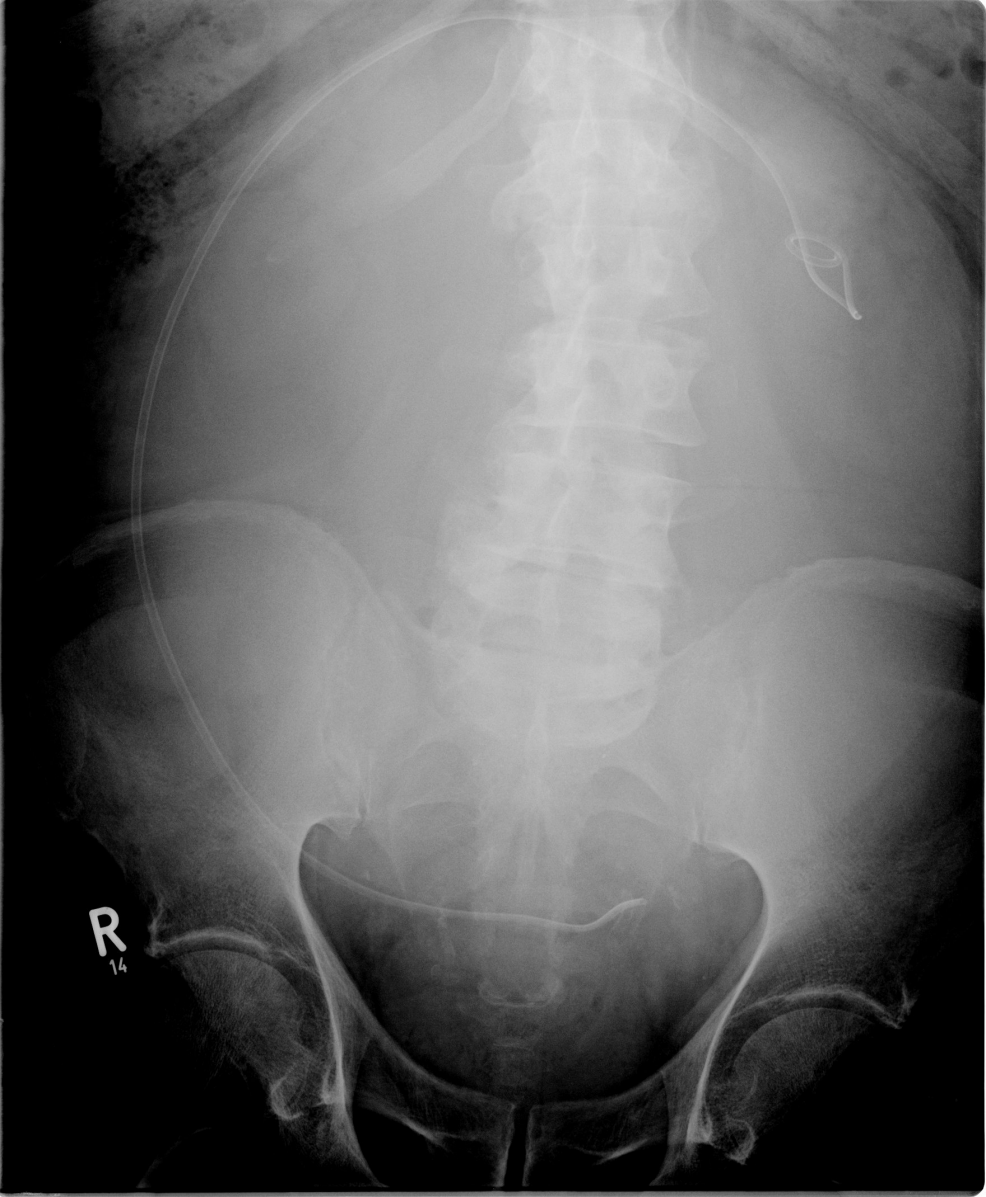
Radiograph after ureter stent insertion.
